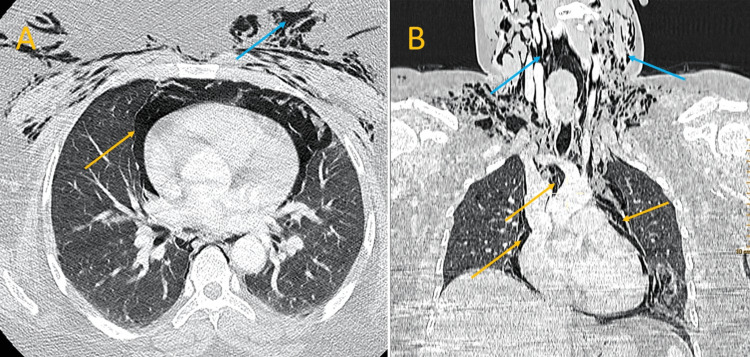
Injected thoracic CT scan in axial section (A), along with sagittal section (B), both in parenchymal window revealing extensive subcutaneous emphysema involving perivascular soft tissues and muscles of the cervical region (blue arrows), as well as massive pneumomediastinum (blue arrows) with esophageal filling
